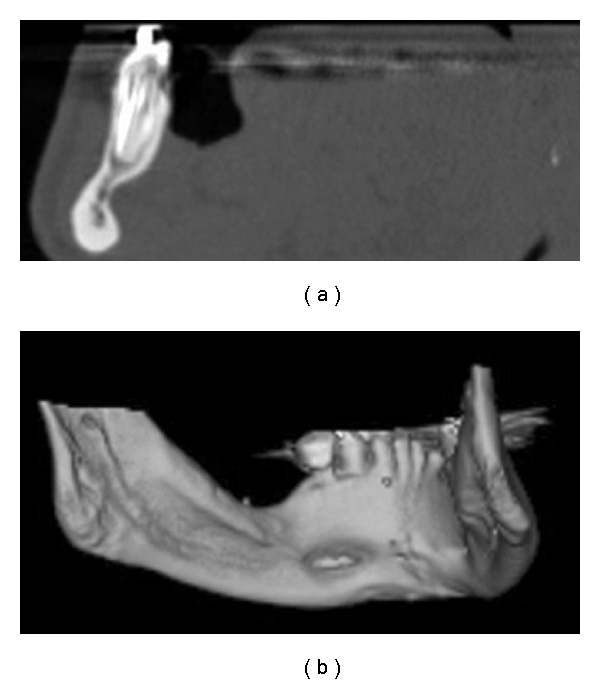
Sagittal computed tomography slices and bone window (a) and 3D reconstruction (b), showing SBD, anterior variant, left side.
